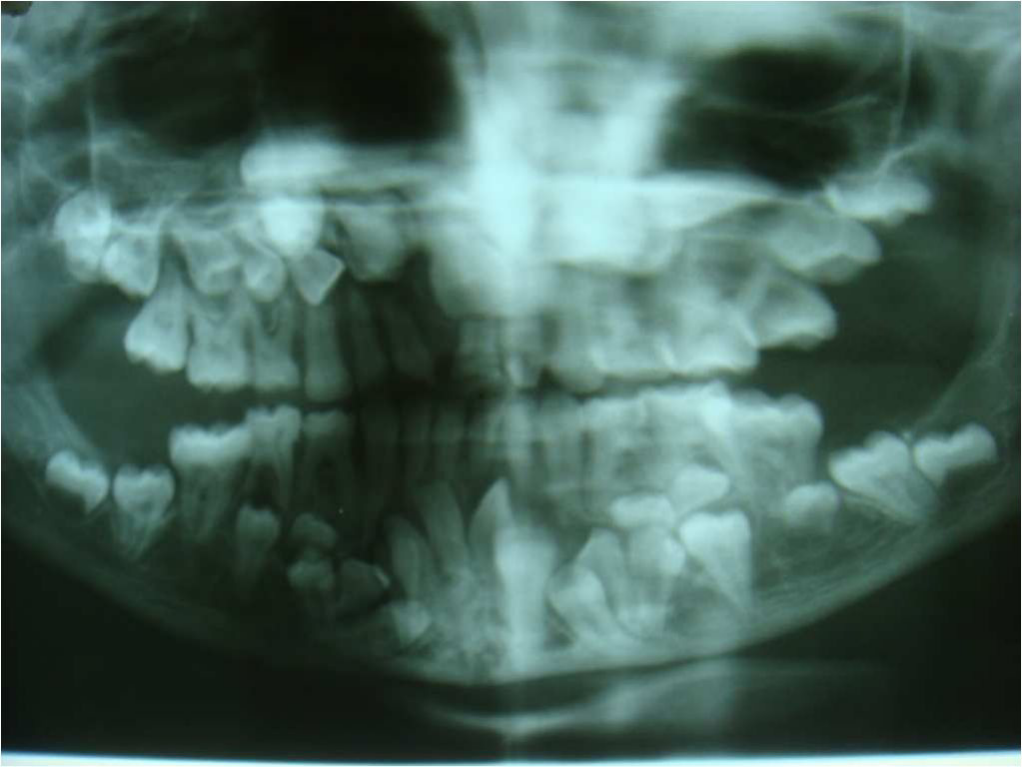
Orthopantomogram showing impacted supernumeraries.
